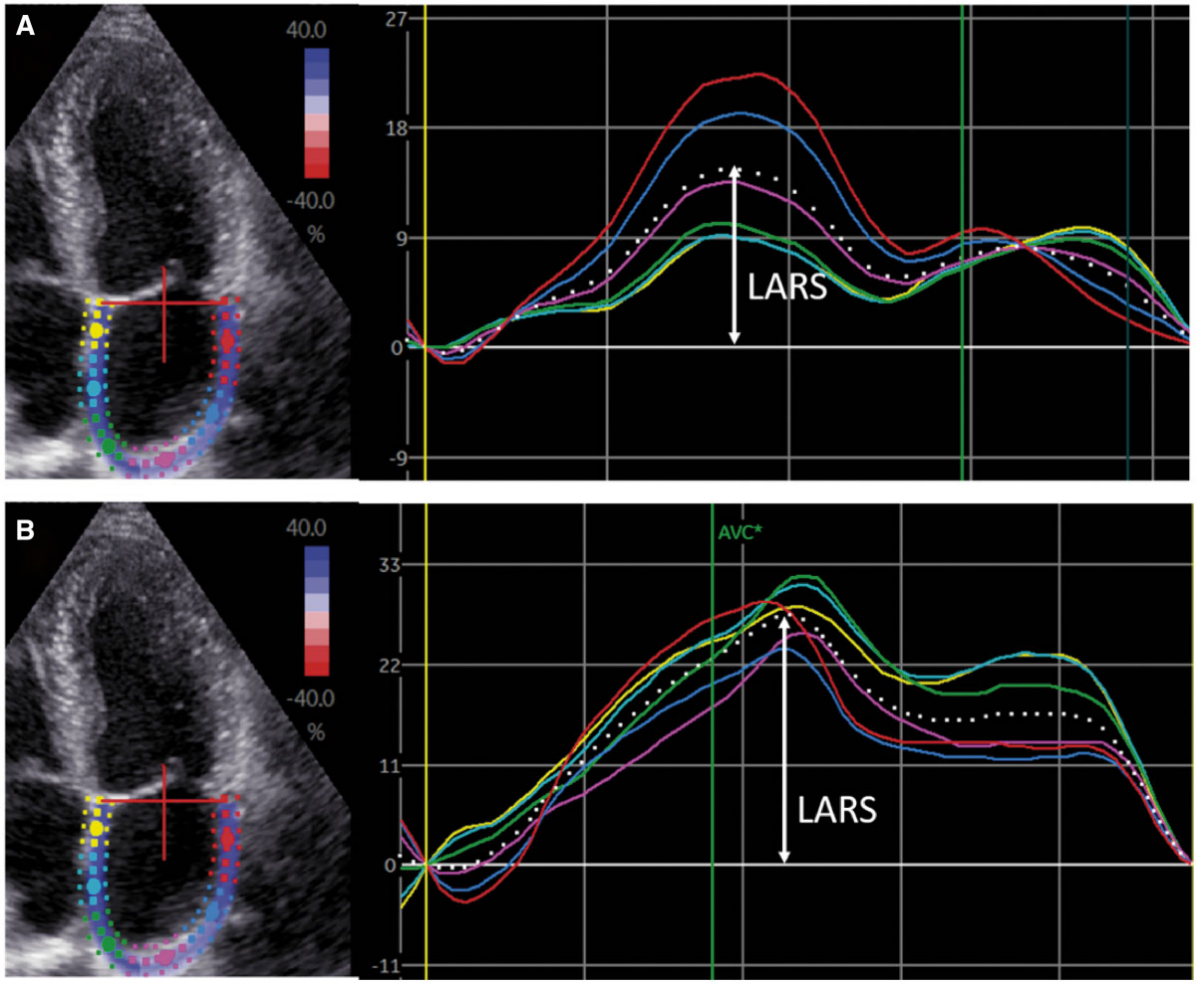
Left atrial speckle tracking strain analysis. LA speckle tracking strain analysis in CRT recipients was performed in an apical four-chamber view. Regional strain vs. time curves are represented by coloured lines, while the average LA strain vs. time curve is represented by the dotted line. LARS is indicated by the arrow. (*A*) Shows the LA strain before CRT implantation (LARS 15%), whereas (*B*) shows the LA strain after CRT implantation (LARS 27%). CRT, cardiac resynchronization therapy; LA, left atrial; LARS, left atrial reservoir strain.
